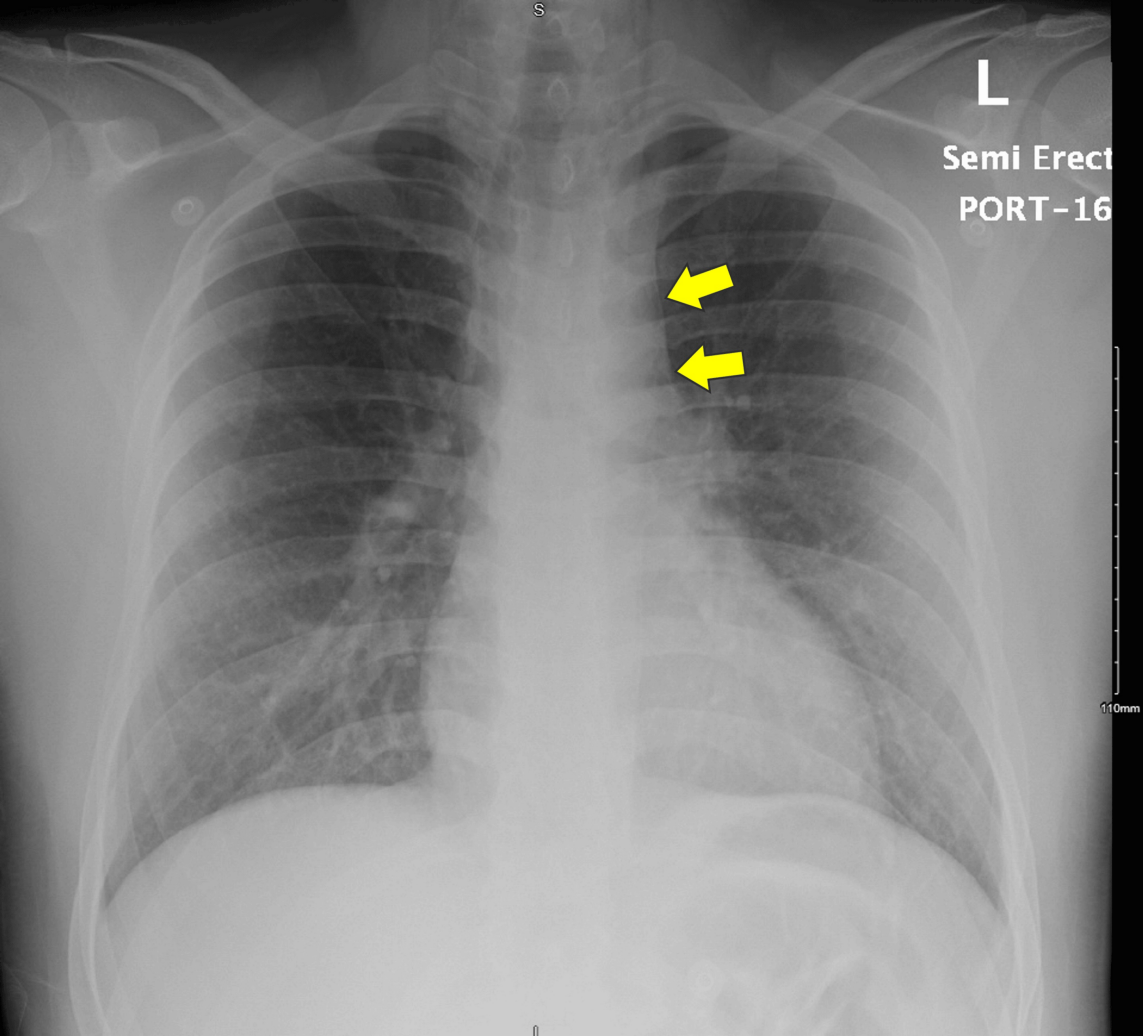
Portable chest radiograph showing pneumomediastinum (arrows)
